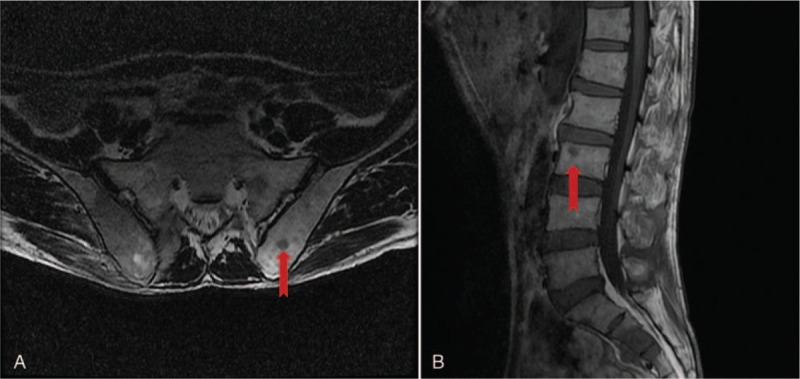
Distant metastasis was shown on his readmission at July 18th, 2019. A. The MRI images showed osteolytic lesions in the ilium. B. Another metastatic lesion was noticeable in lumbar vertebra (indicated by arrows).
